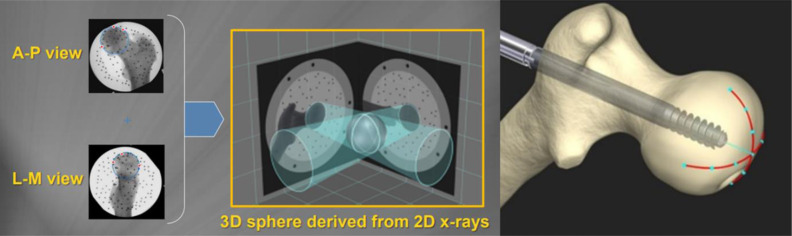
Principle:* A 3D model is constructed by analyzing position markers obtained from 2D X-ray images in two directions. *Concept of TSD*: TSD is the distance from the tip of the screw to the surface of the femoral head.
